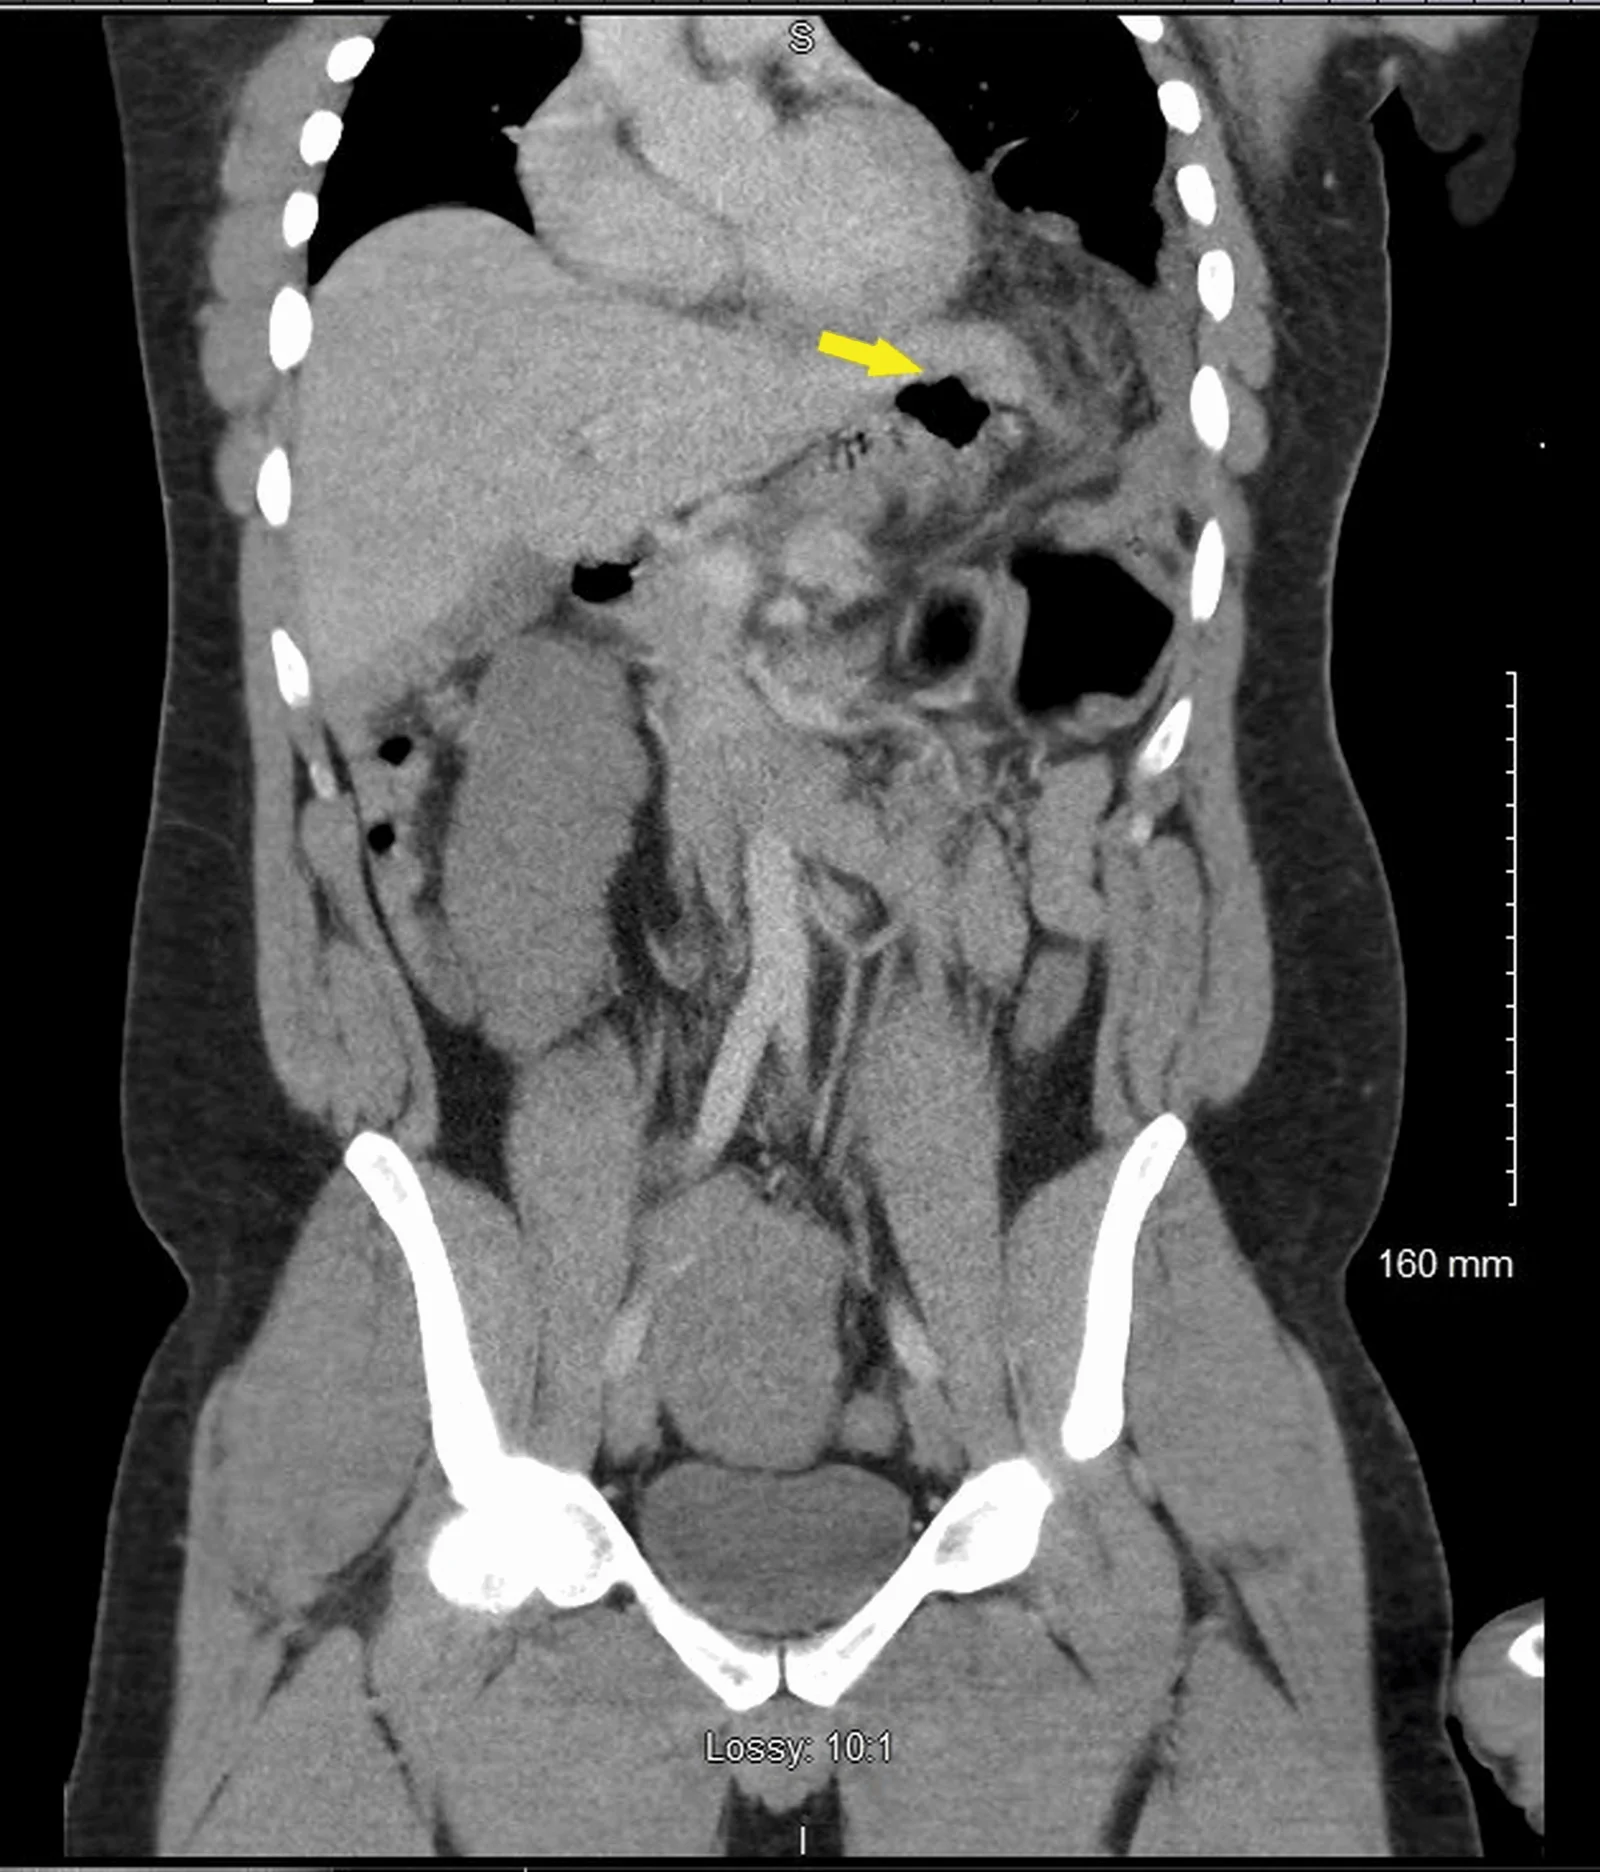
Preoperative contrast-enhanced computed tomography. The image demonstrates a left posterolateral diaphragmatic defect (yellow arrow) through which the splenic flexure and omental fat have herniated. The transition point and adjacent inflammatory changes are consistent with mechanical obstruction and raise concern for incarceration.
